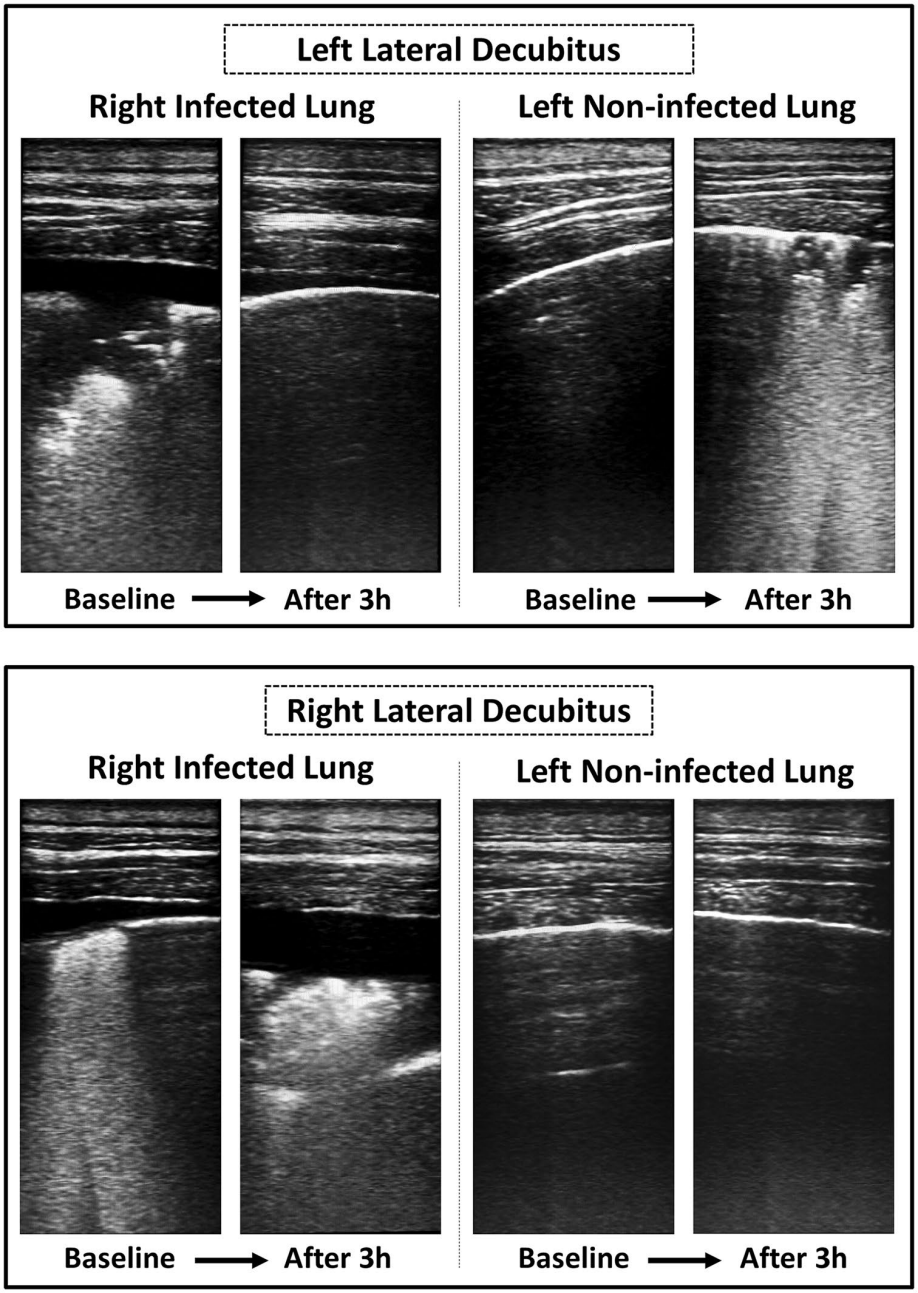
Lung Ultrasound images. In the upper section, a decrease in lung aeration is observed in the non-infected lung when dependent. In contrast, the infected lung (non-dependent) shows some re-aeration. In the lower section, lung ultrasound worsens further for the infected dependent lung.
